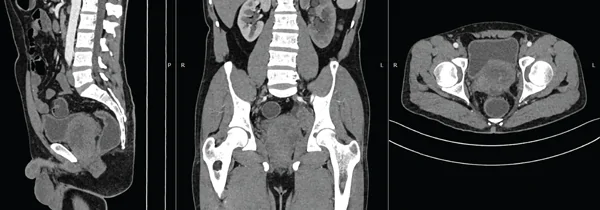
Contrast‐enhanced CT (CECT) of the abdomen and pelvis at diagnosis (November 2024), in sagittal, coronal and axial planes. A grossly enlarged, lobulated prostate mass (6.5 × 6.0 × 5.5 cm) causes bladder outlet obstruction and left hydronephrosis. Enlarged pelvic lymph nodes are present.
